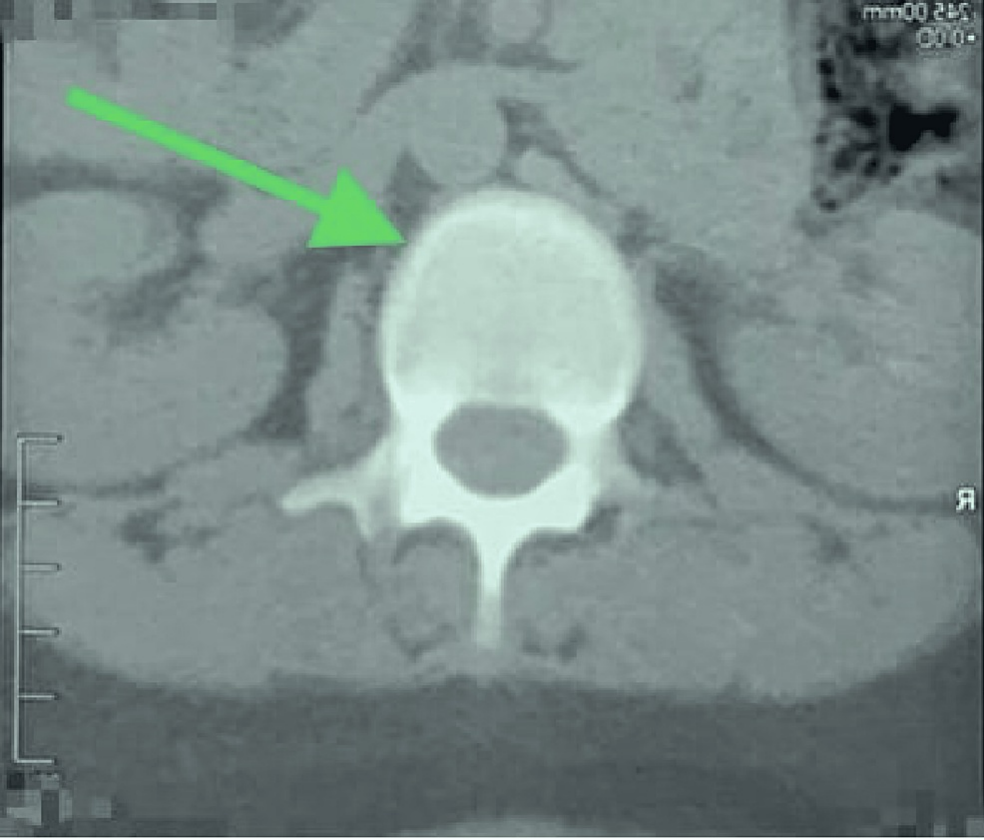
CT scan of the spine in the coronal plane view shows resolution of the intraosseous air foci at the L3, as indicated by the arrow. CT: computed tomography, L3: third lumbar spine vertebra
